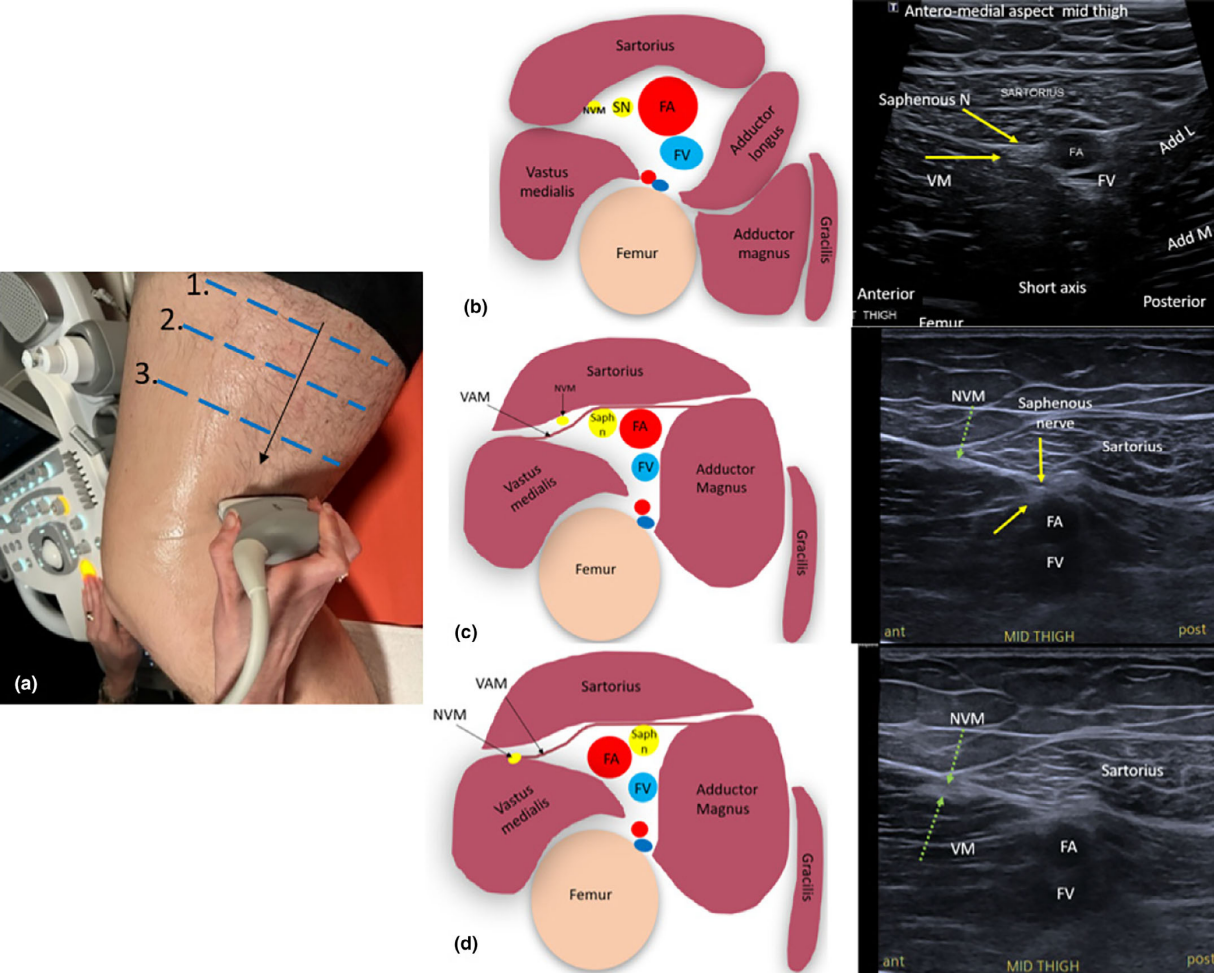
Sonographic imaging and anatomy of the saphenous nerve (Saph n) and nerve to the vastus medialis (NVM) within the adductor canal at three locations from proximal to distal. (a) Photo indicating transducer positions for short‐axis imaging of the saphenous nerve within the adductor canal. Figures (b), (c) and (d) correspond to transducer positions 1, 2 and 3 (blue lines) in the photo in (a). The NVM (green dashed arrows) is located anterior to the saphenous nerve and superficial to the vasto‐adductor membrane (VAM). On axial sonographic sweeps from proximal to distal, the saphenous nerve (yellow arrows) lies deep to the sartorius muscle and appears to cross superficially to the femoral artery (FA) in an anterior to posterior direction. Add L, adductor longus muscle; Add M, adductor magnus muscle; ant, anterior aspect of image; FA, femoral artery; FV, femoral vein; post, posterior aspect of image; SN, saphenous nerve; VM, vastus medialis muscle.
